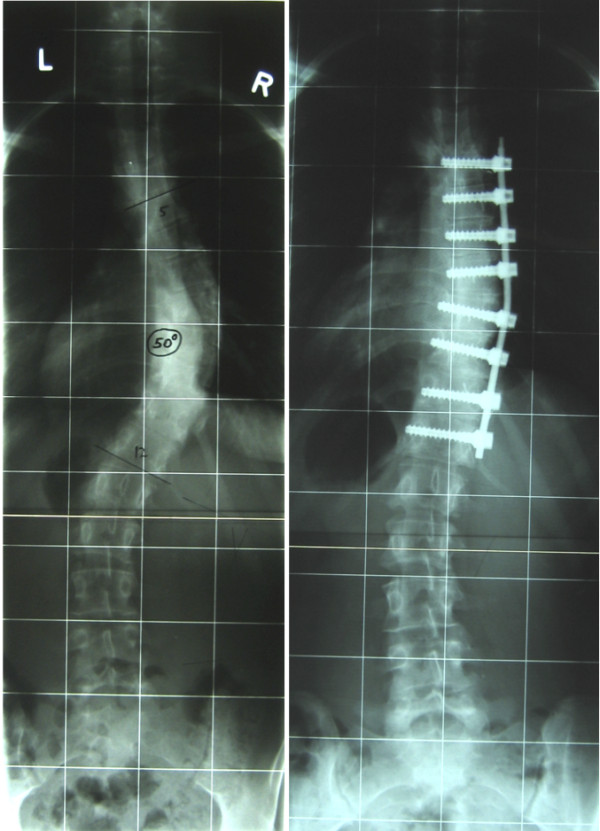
Preoperative curve of 50° on AP radiograph (left) and postoperative curve of 20° (right).
